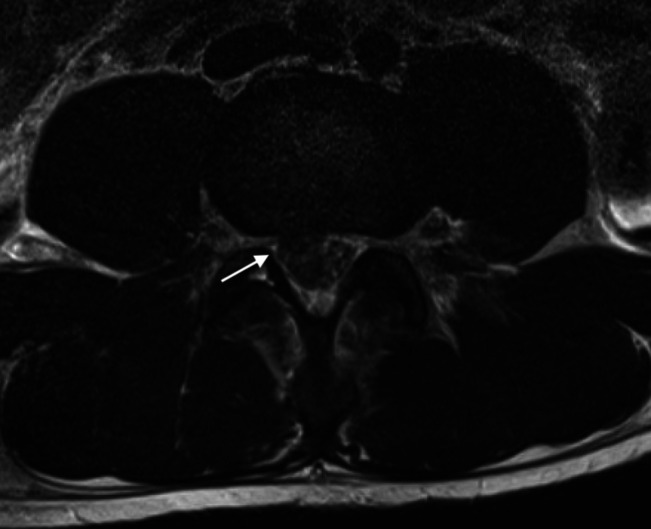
Axial MRI cut at L3-4 showing reported disk extrusion.
